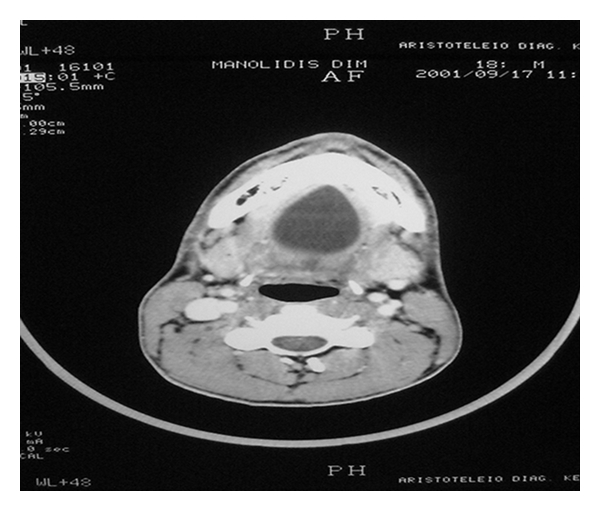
Section of computed tomography (CT) showing a sizeable cystic formation with distinct boundaries.
